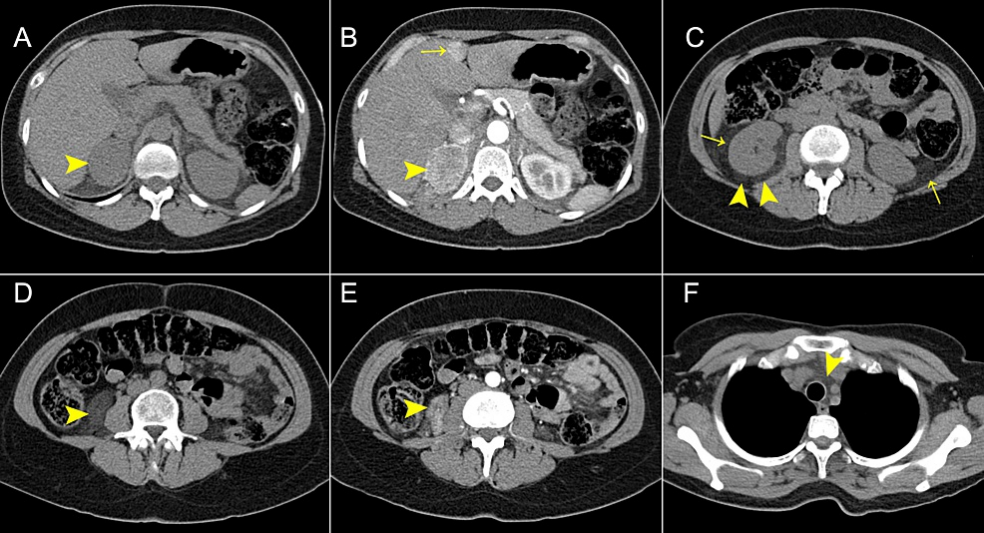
CTA of the thoracoabdominal aorta. (A) The NECT axial plane shows a right-sided, round, and well-defined adrenal mass (➤) without calcifications or fat areas. The mass measured 41 x 46 x 34 mm. (B) In the CECT arterial phase, the adrenal mass showed avid and heterogeneous enhancement up to 145 HU (➤). Incidental hypervascular liver nodules were seen (→). (C) NECT of the perinephric bilateral spaces with striated fat (→) and pseudonodular lesions (➤), mainly to the right side. (D) Below the right renal pole was the bigger pseudolesion that measured 21 x 44 x 32 mm (➤) and had attenuation rounding of -51 HU in the NECT. (E) This nodule had enhancement with the contrast agent (➤). (F) NECT with striated fat in the mediastinum (➤). The measurement units are in millimeters (mm) and are in order length x anteroposterior x transverse. CTA: computed tomography angiography; NECT: non-enhanced computed tomography; CECT: contrast-enhanced computed tomography; HU: Hounsfield unit.
